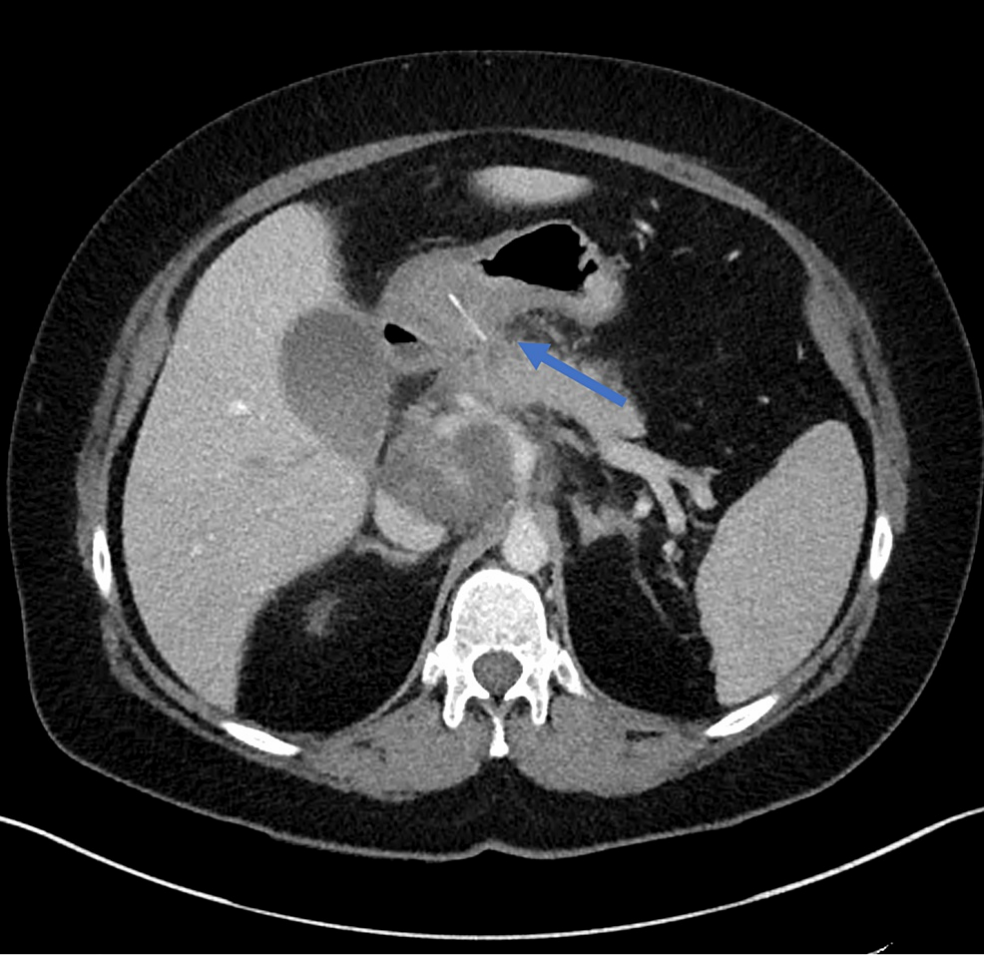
CT of the abdomen and pelvis with portal venous contrast axial view on initial patient presentation. A foreign body is indicated by the arrow.
